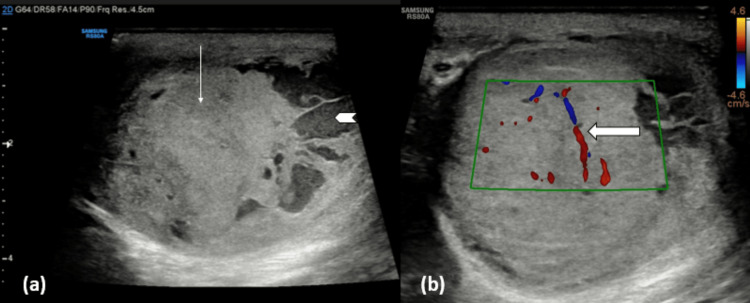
Ultrasonography scrotal image (a) showing a heterogenous lesion with solid (line arrow) and cystic (chevron arrow) components in the right testis and (b) raised vascularity of the testicular lesion (block arrow).
